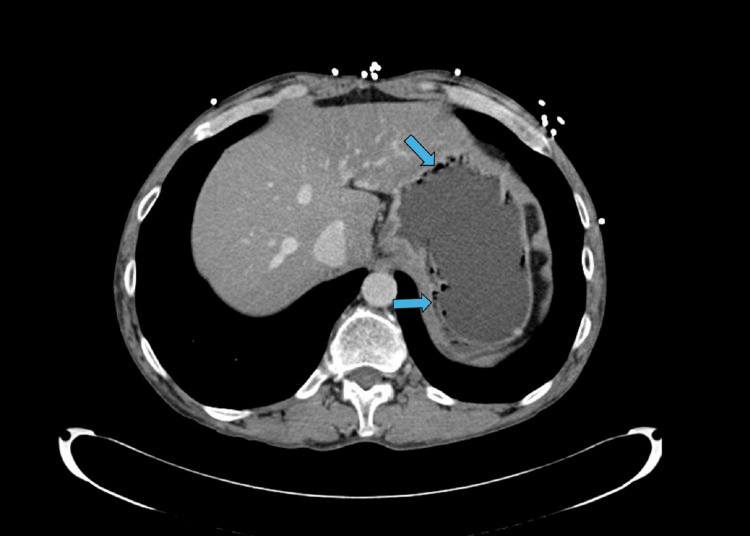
Computed tomography scan of the abdomen (axial view) The image shows marked distention of the fluid-filled stomach. Blue arrows indicate tracking along the stomach concerning gastric pneumatosis.
